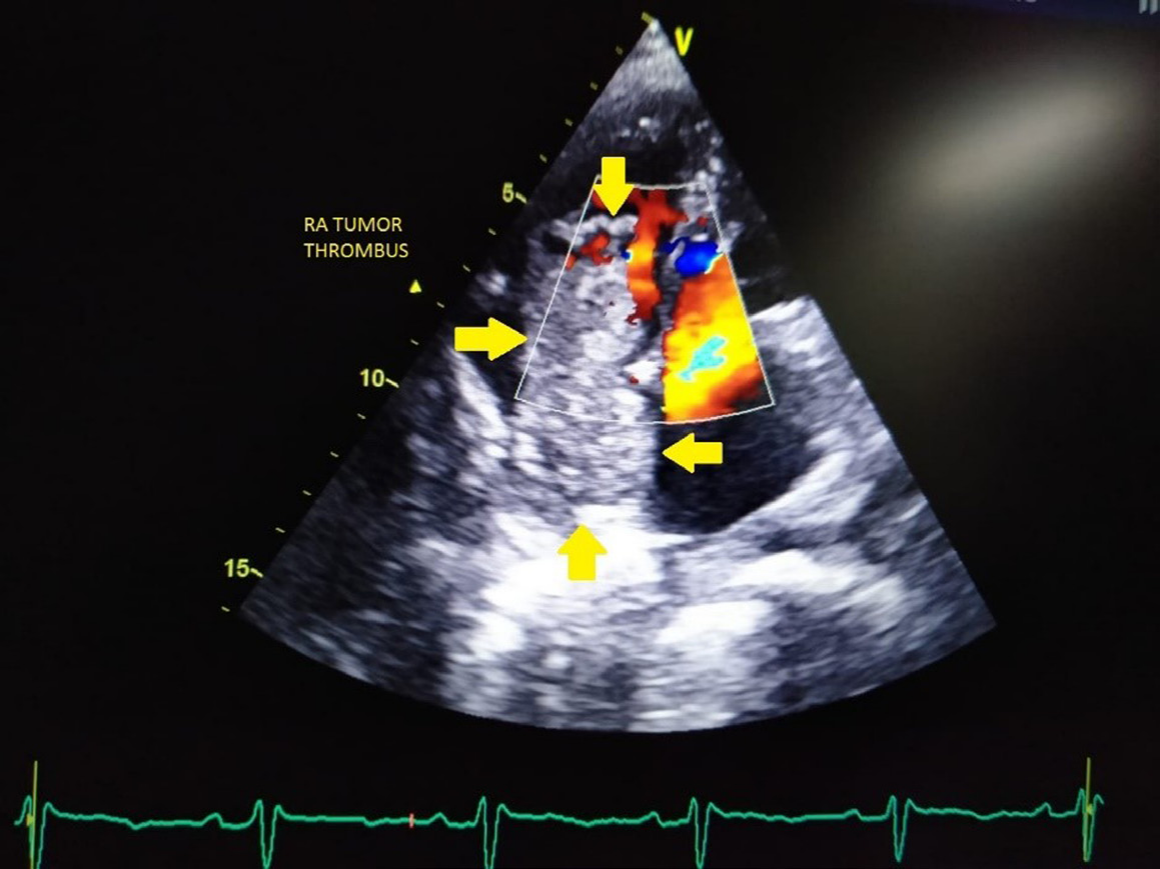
Transthoracic echocardiography image showing a large intracardiac right atrial thrombus measuring 5.7 × 2.4 cm obstructing the tricuspid valve during the diastolic phase of the cardiac cycle (RA; arrow).
